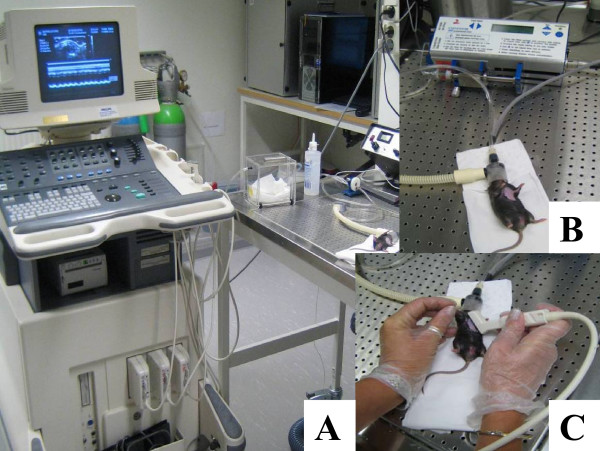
A-C. Experimental setup. A. The echocardiographic system. B. Anesthetic setup. C. The high-frequency 15-MHz linear transducer.
